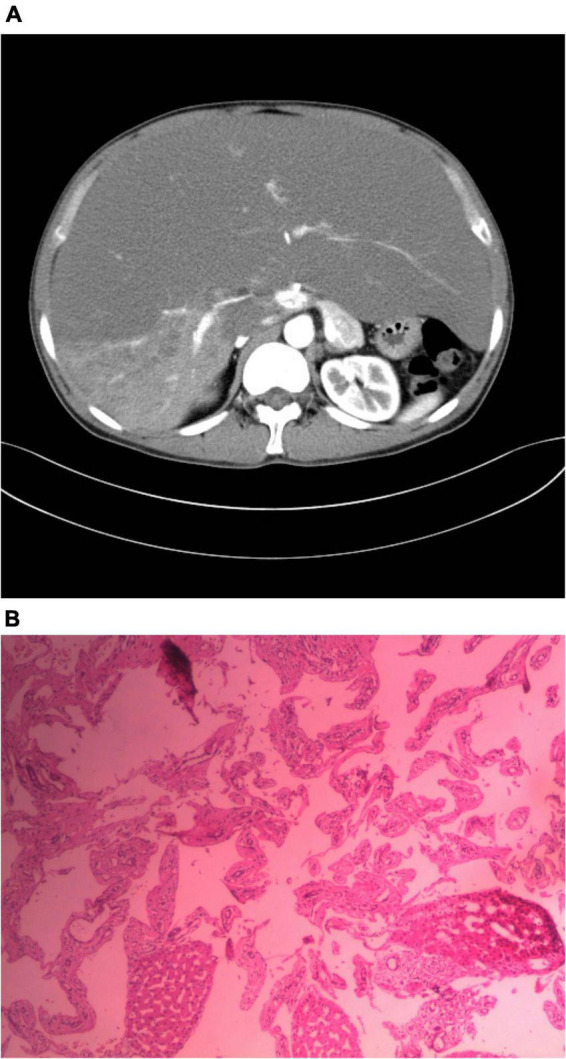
(A) CT scan of the lesion. (B) Pathological images.
